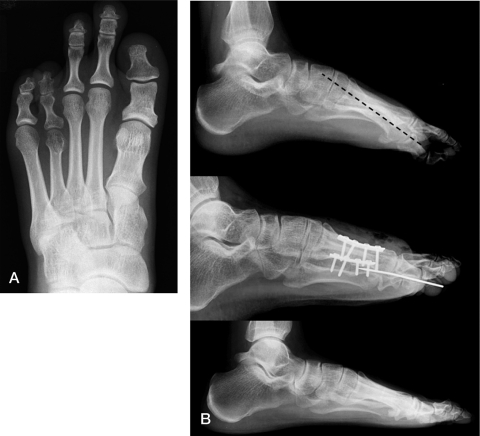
A. Shortening of the first and fourth metatarsals. B. Lateral radiography after consolidation of lengthened metatarsal bone showed cavus deformities (top, dotted line) of the first and fourth metatarsals. Dorsal closing wedge osteotomies were performed (middle) and the final radiograph (bottom) shows correction of cavus deformity.
